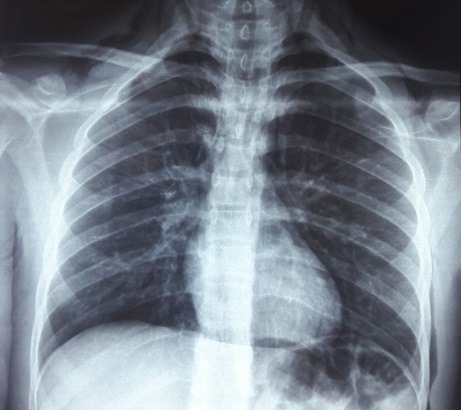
Radiographie thoracique montre un pneumomédiastin, un emphysème des parties molles cervico-thoraciques avec le signe de diaphragme continu
